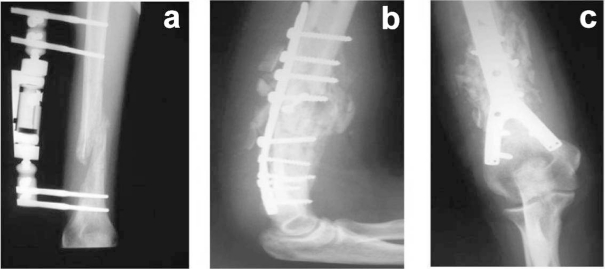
(a) AP x-ray demonstrating the absence of callus formation; (b, c) Postoperative AP and lateral x-rays after osteosynthesis of the fracture by means of a 'Y' plate non-union of the fracture after the end of antibiotic therapy and internal fixation of the non-union of the humerus.
